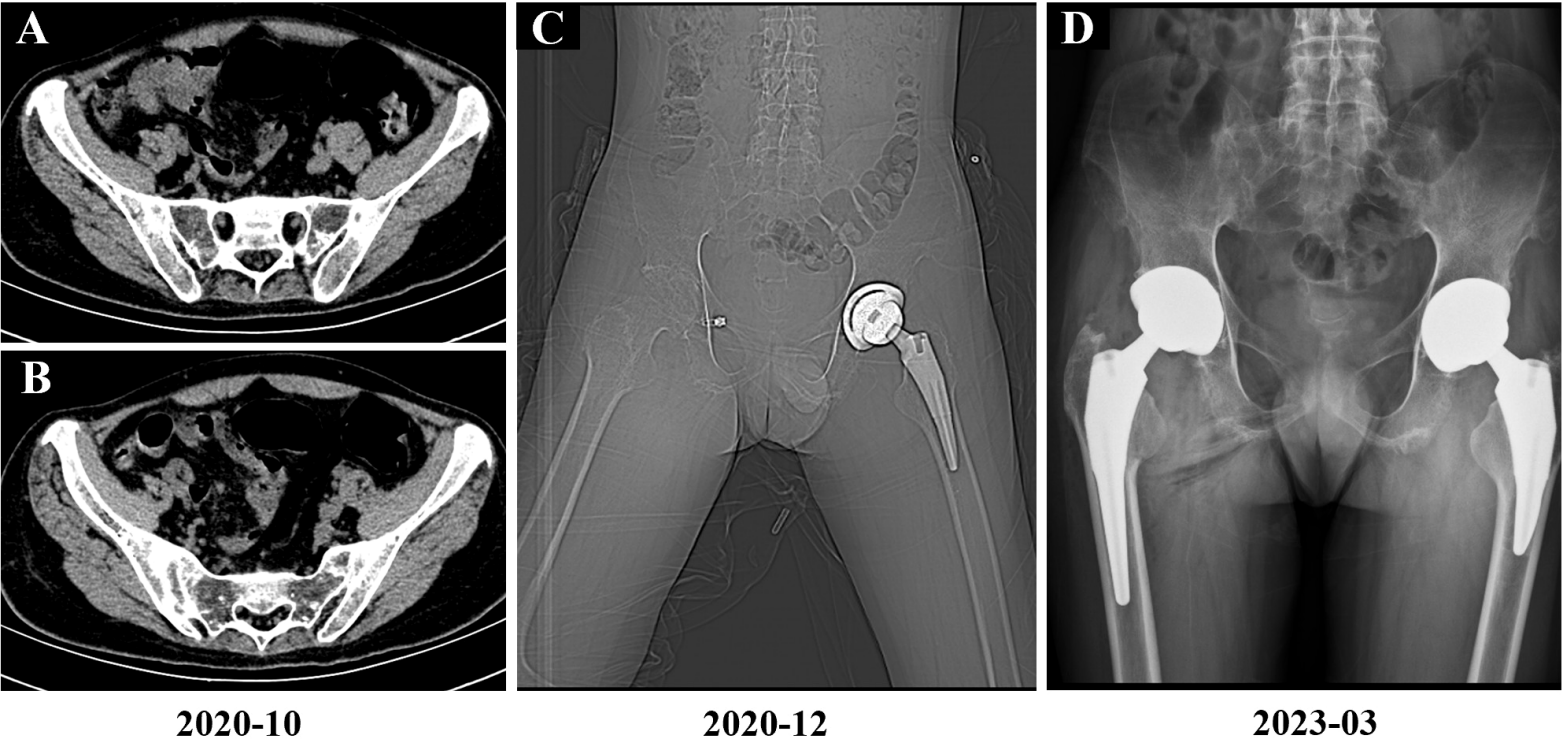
Imaging findings of the patient. (A, B) October 2020 sacroiliac joint CT demonstrating bilateral bony ankylosis with complete obliteration of joint spaces. (C) December 2020 postoperative imaging following left total hip arthroplasty (CT localizer image). (D) March 2023 postoperative imaging following right total hip arthroplasty (anteroposterior radiograph).
